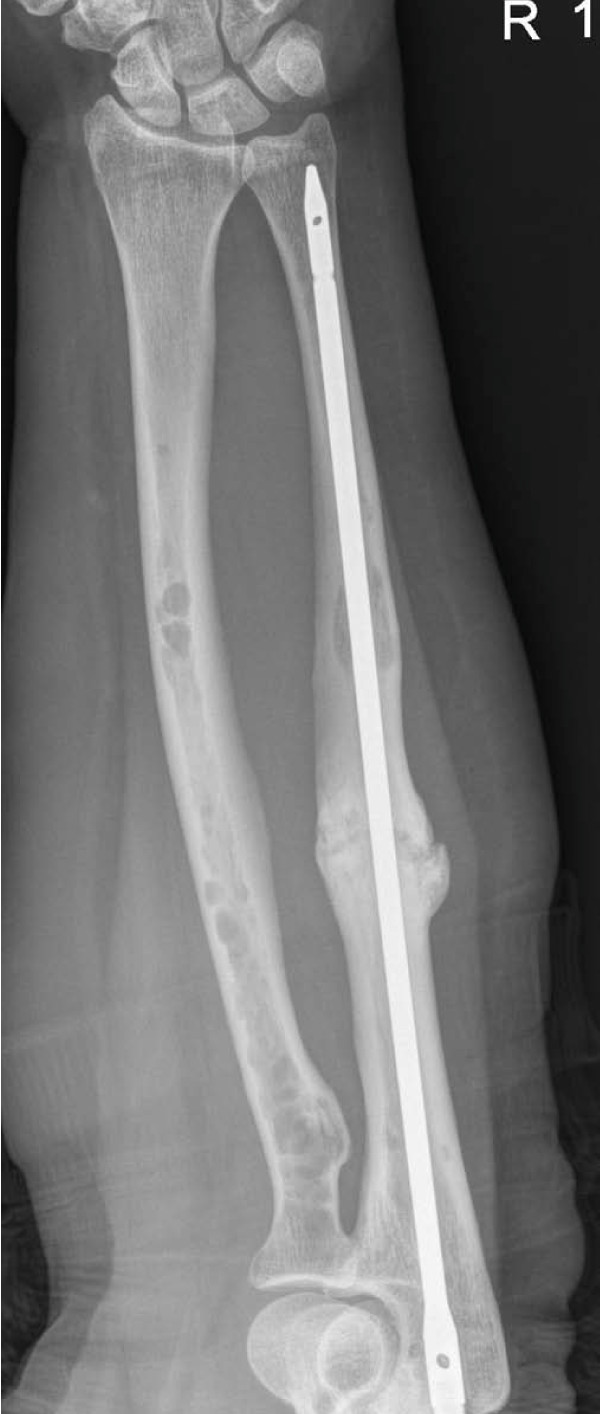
Radiographic union of pathological fracture of right ulna at 12 months post-operatively.
